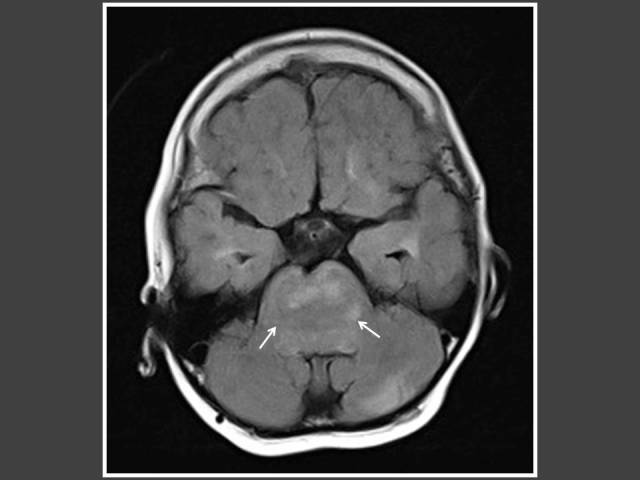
Seven years-old girl who presented with bilateral breast enlargement. T2-FLAIR axial MRI of the brain shows brainstem glioma (arrows).
